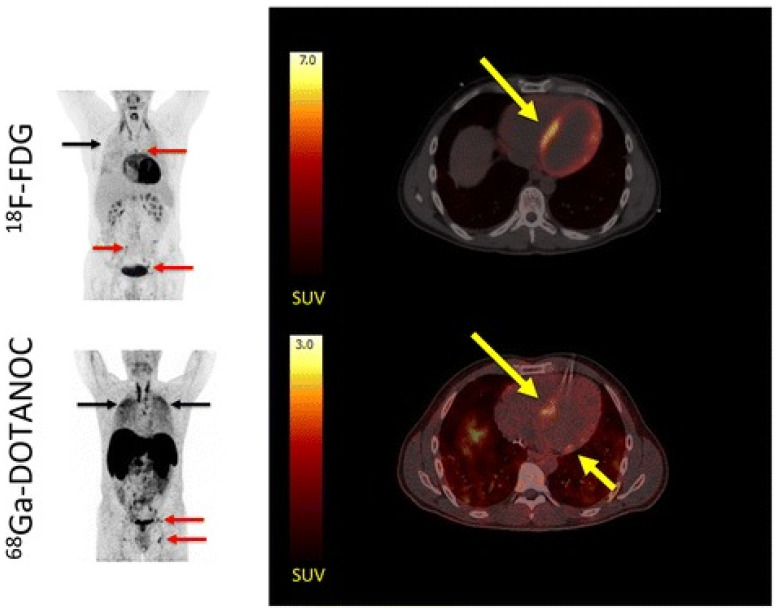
Comparative maximum intensity projection (MIP) and transaxial PET/CT images of ^18^F-FDG (upper row) and ^68^Ga-DOTANOC (lower row) in a patient with suspected cardiac sarcoidosis (adapted from Gormsen et al., 2016 [23], licensed under CC-BY 4.0). (Left) MIPs show avid hilar lymph node uptake (red arrows) and pulmonary parenchymal activity (black arrows) on both tracers, indicating systemic sarcoidosis involvement. (Right) Transaxial slices reveal focal-on-diffuse myocardial ^18^F-FDG uptake (SUV_max_ = 21; yellow arrow), which was rated inconclusive by most reviewers because of incomplete physiological suppression. In contrast, ^68^Ga-DOTANOC shows distinct focal septal uptake (SUV_max_ = 2.8; target-to-background = 3.0; yellow arrow), rated pathological by all reviewers. These images demonstrate the improved lesion conspicuity and reduced myocardial background achievable with SSTR-targeted imaging compared with FDG, facilitating diagnosis of cardiac involvement in sarcoidosis.
